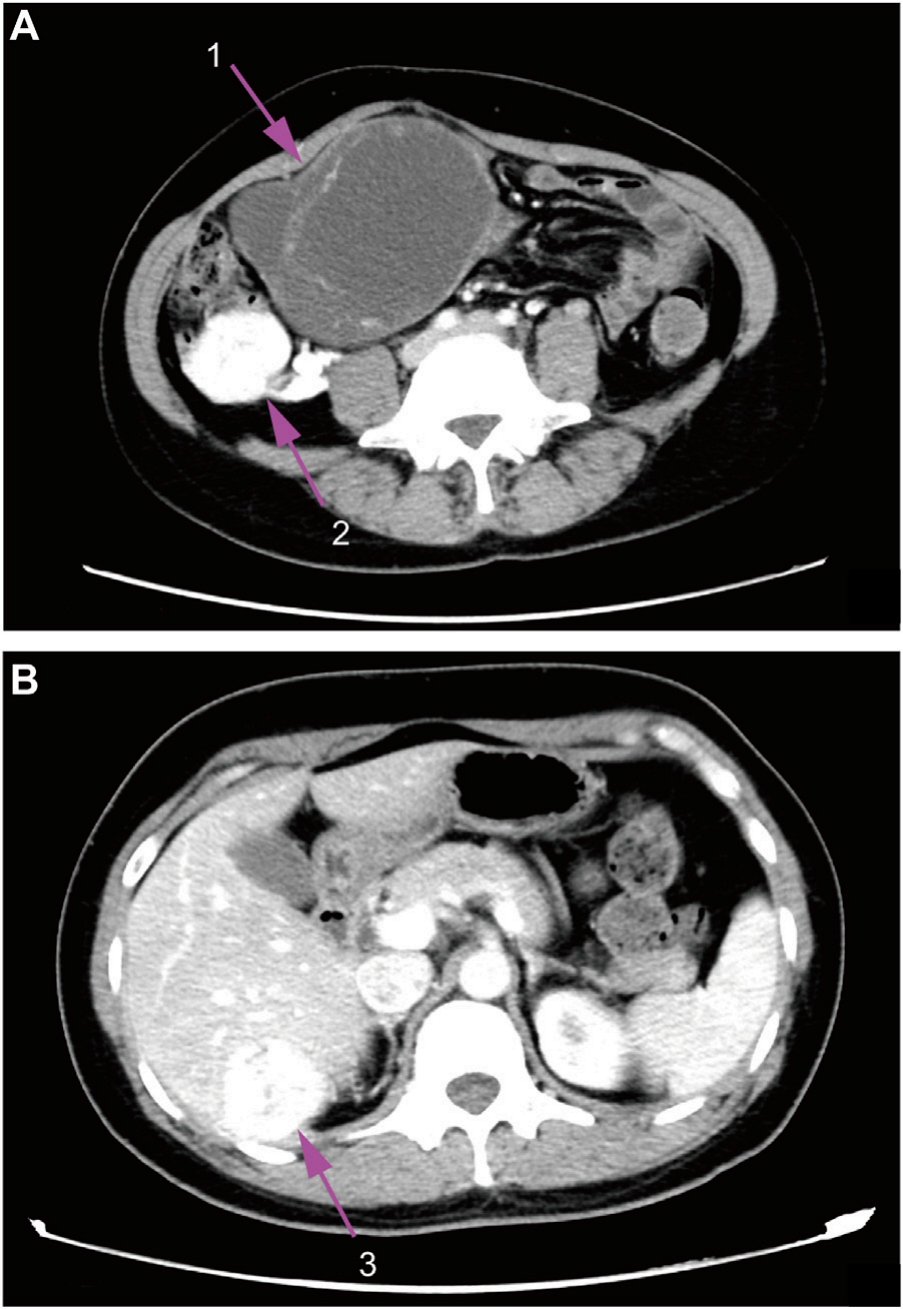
Contrast-enhanced CT images. (A), abdomen image. (B), pelvic cavity image; 1, ovarian mass; 2, surrounding lesions of the ovarian mass; 3, enhancing liver mass.
